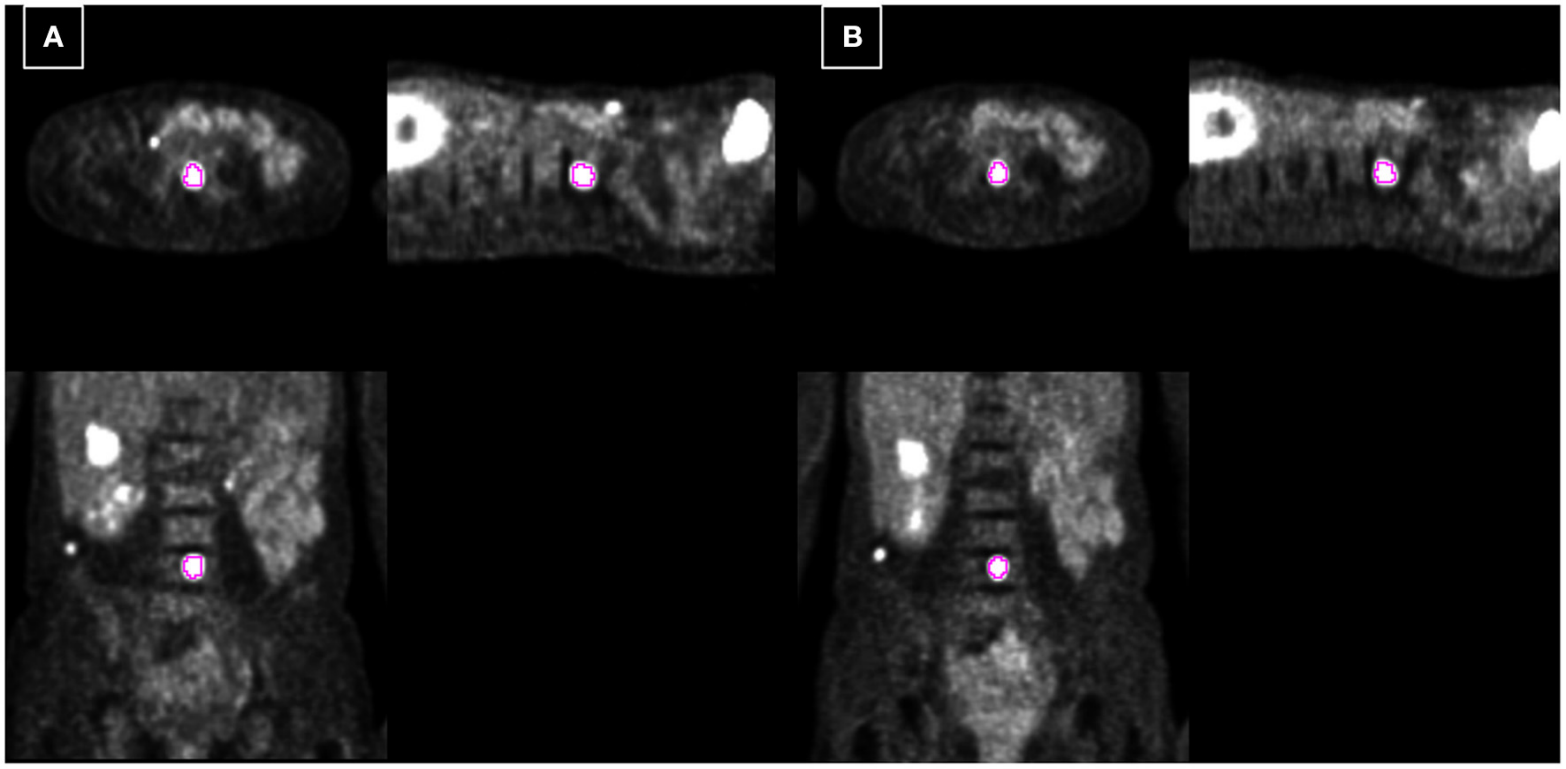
Example 2: 54-year-old female with spine metastasis of melanoma. Each PET-CT (A) and PET-MR (B) was acquired 73 and 48 min after injection. The tumor is delineated based on fixed 40% threshold to SUVmax.
